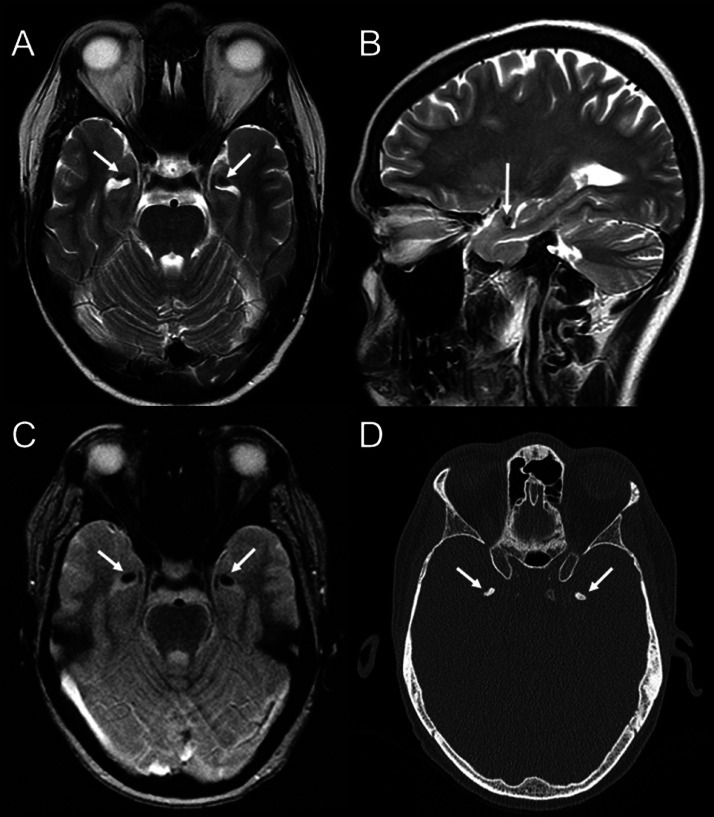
Axial (A), sagittal (B) T2-weighted, and axial T2* (C) images demonstrate bilateral hypointensities (arrows) in amygdalae in a 42-year-old male patient with lipoid proteinosis. (D) An axial CT image confirms calcifications.
